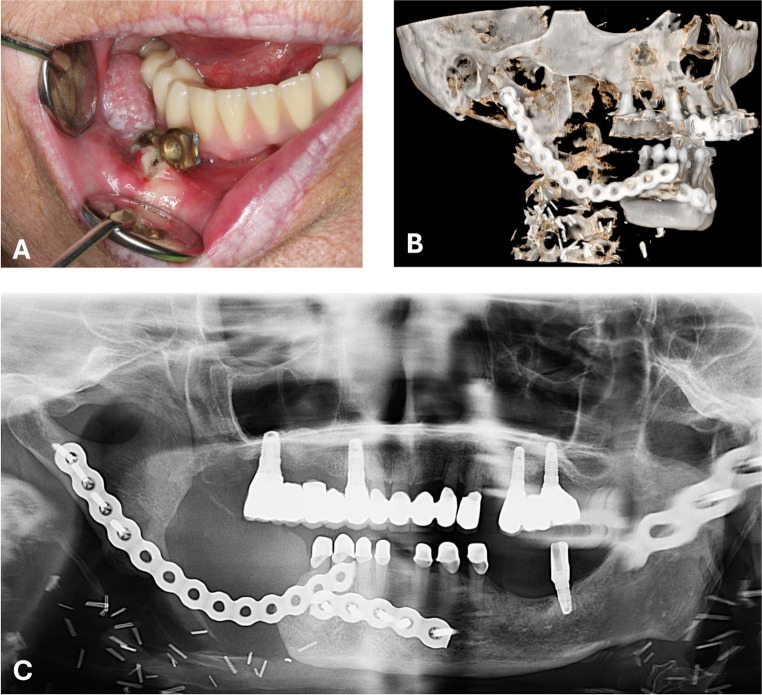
Fractured RP, penetrating the oral cavity, underlining the necessity for removal (A), radiographic images (B: virtual reconstruction of a Cone Beam Computed Tomography (CBCT), C: panoramic radiograph) of the same patient
